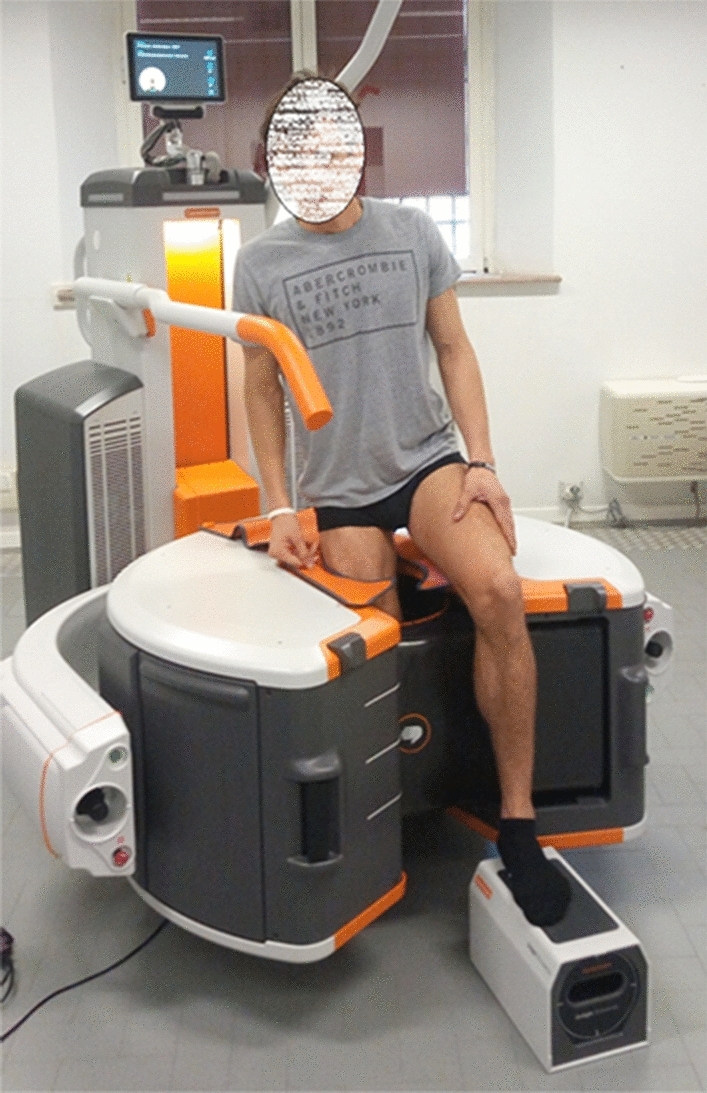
Picture taken from one typical patient during scan with the CBCT in weight-bearing
